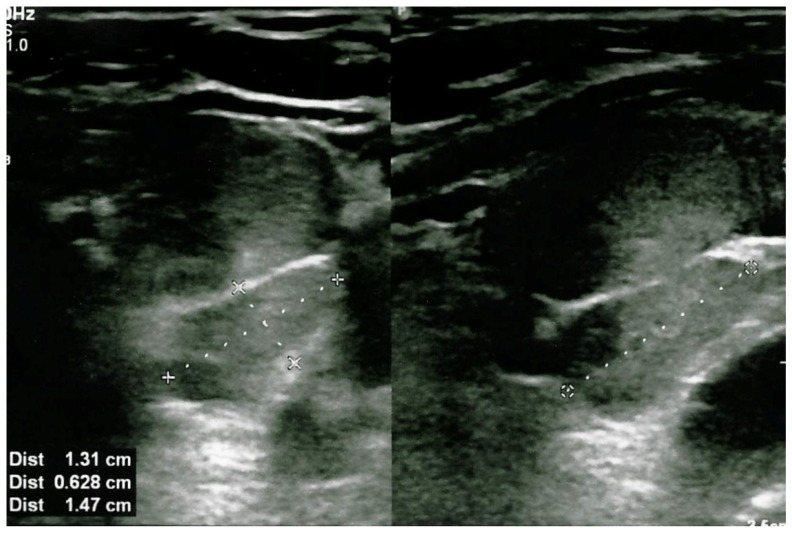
The ultrasonography revealed a well-defined, hypoechoic, homogeneous lesion measuring 13 × 6 × 15 mm, located inferior to the lower pole of the left thyroid lobe, suggestive of a parathyroid adenoma, prior to fine-needle aspiration biopsy (FNAB).
